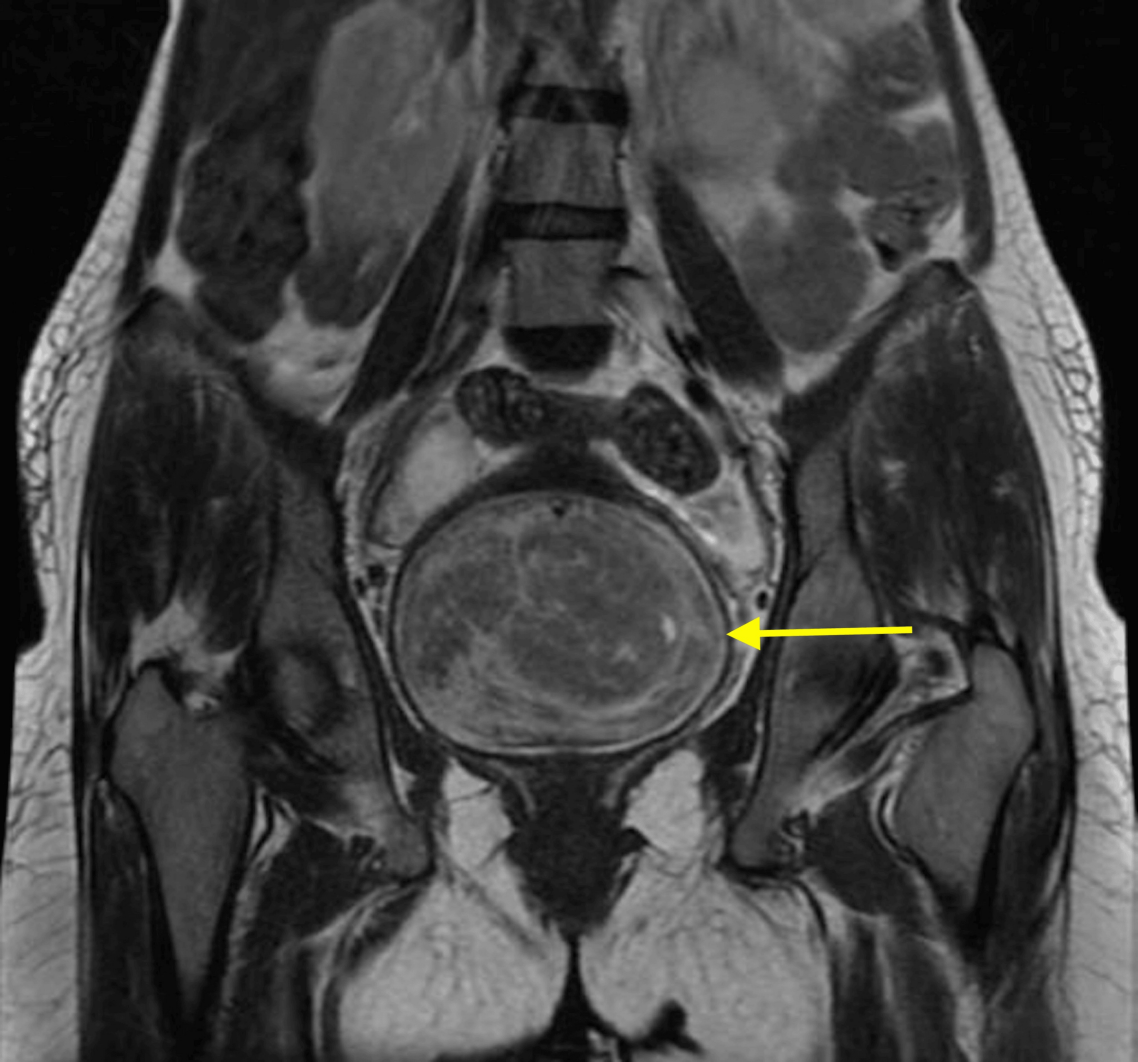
Magnetic resonance imaging (MRI) with yellow arrow pointing at a large well defined heterogeneous altered signal intensity lesion measuring 11.5 × 9.5 × 9.9 cm
